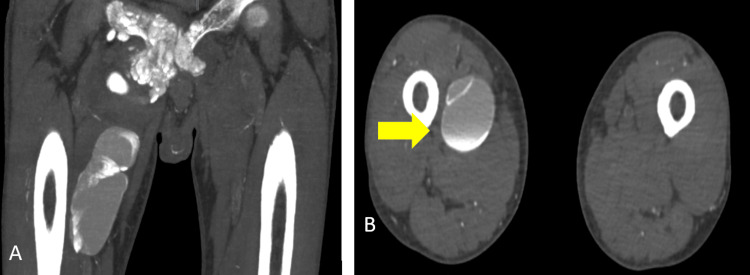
Plain CT scan of the lower extremities Large cystic mass with fluid-calcium layering or sedimentation sign in the right upper inner thigh measuring 11.6x4.3x4.6 cm (CCWAP). CT: computed tomography
